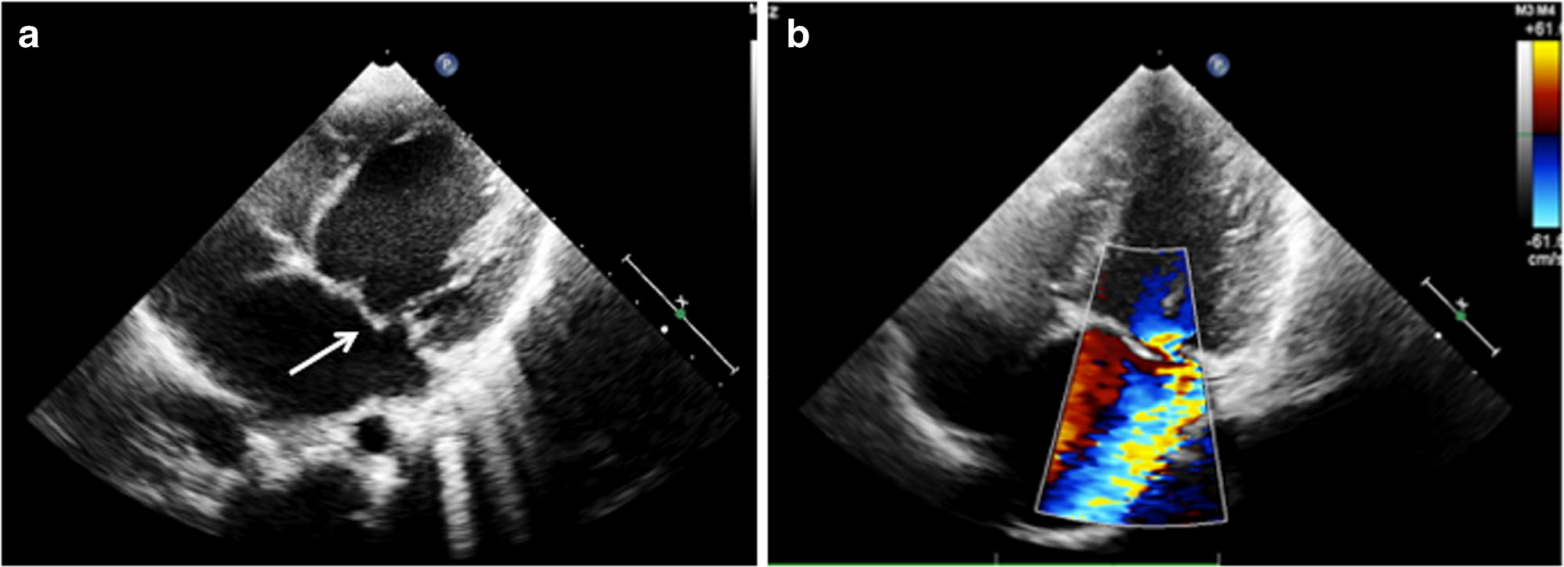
Echocardiogram from a patient with severe mitral regurgitation secondary to a flail anterior mitral leaflet (*arrow*) (a). Color Doppler shows severe eccentric mitral regurgitation (b).
